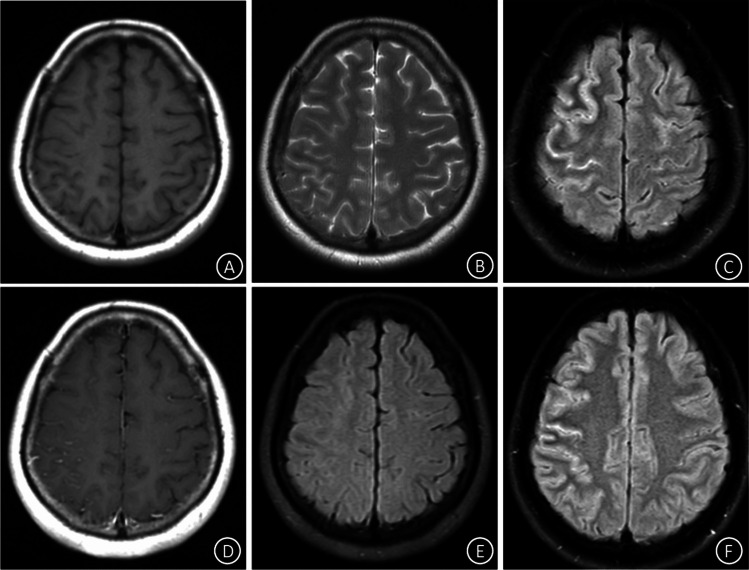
Cranial MRI findings. Upon first presentation, no obvious abnormal signal was seen in the (A) T1 or (B) T2 images. T2-FLAIR showed (C) hyperintensity in the right frontoparietal lobe and (D) spot enhancement. One month later, the high FLAIR signal had disappeared (E). Upon recurrence, the high FLAIR signal in right frontoparietal lobe reappeared (F)
